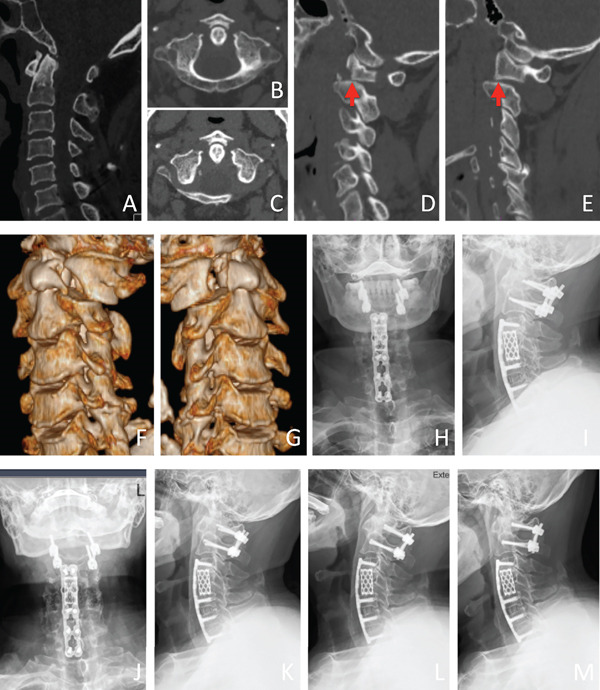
Case Example (transverse ligament injury): (A) CT sagittal reconstruction shows normal atlantodental interval, reduced basion‐dontoid distance and concurrent posterior dislocation of atlas/occiput. (B–C) Axial CT reveals anterior arch fracture at the arch‐lateral mass junction with odontoid displacing anteriorly carrying an avulsed fragment, plus transverse ligament insertion avulsion fracture (arrows). (D–G) Sagittal/3D reconstructions demonstrate bilateral anterior arch fractures with posterior C1–C2 displacement (red arrows). (H–I) One‐year postoperative radiographs confirm proper occipitocervical alignment and hardware position. (J–M) Four‐year follow‐up radiographs (AP/lateral/dynamic flexion‐extension views) show intact instrumentation without loosening/fracture and solid fusion mass formation.
